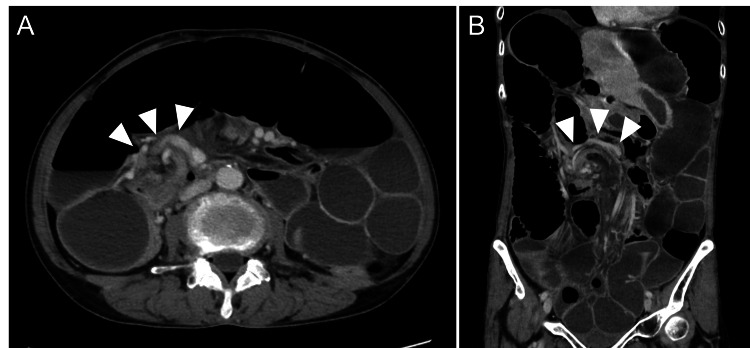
CT showing small bowel volvulus (A) Axial CT image showing the whirlpool sign of the mesentery (arrowheads), indicating mesenteric torsion. (B) Coronal CT image showing the same finding (arrowheads) CT: computed tomography
